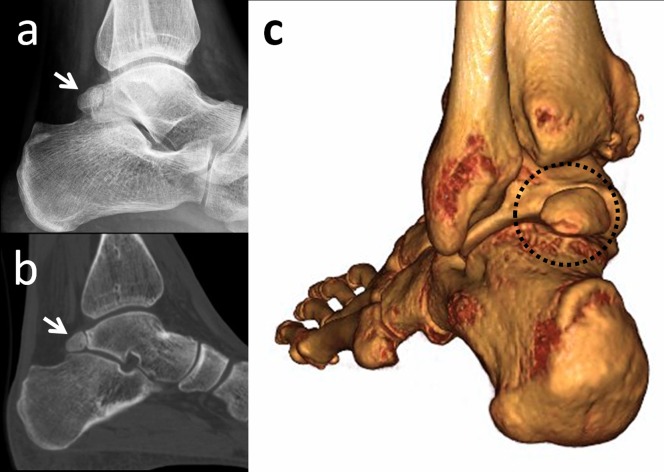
An os trigonum is best seen on lateral foot radiographs (a) Lateral foot radiograph; (b) 2D computed tomography (CT) appearance; (c) 3D CT.
